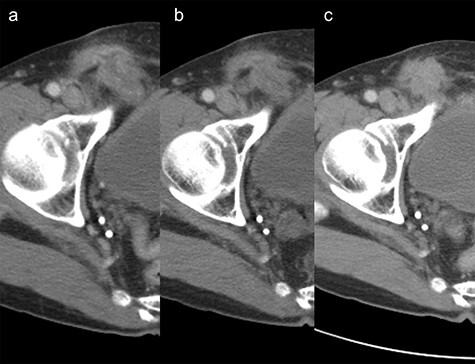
Change over time after transverse colon resection in the right inguinal area on computed tomography scan. (a) 7 years year after hernia repair. (b) 8 years after hernia repair. (c) nine and a half years after hernia repair.
